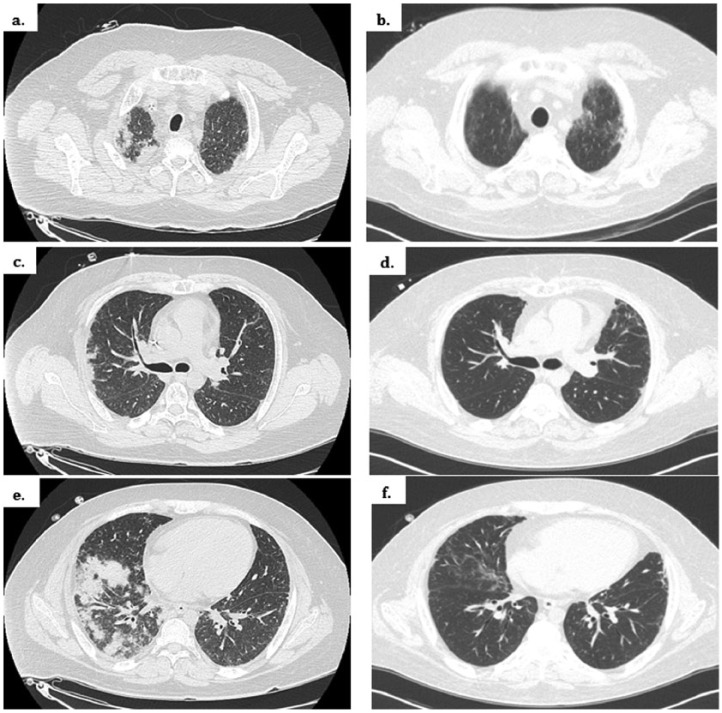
Radiographic comparison before and after corticosteroid therapy. Admission CT scan (left; [a] upper lung zone, [c] middle lung zone, [e] lower lung zone). Two months after high-dose corticosteroid therapy with marked improvement (right; [b] upper lung zone, [d] middle lung zone, [f] lower lung zones).
